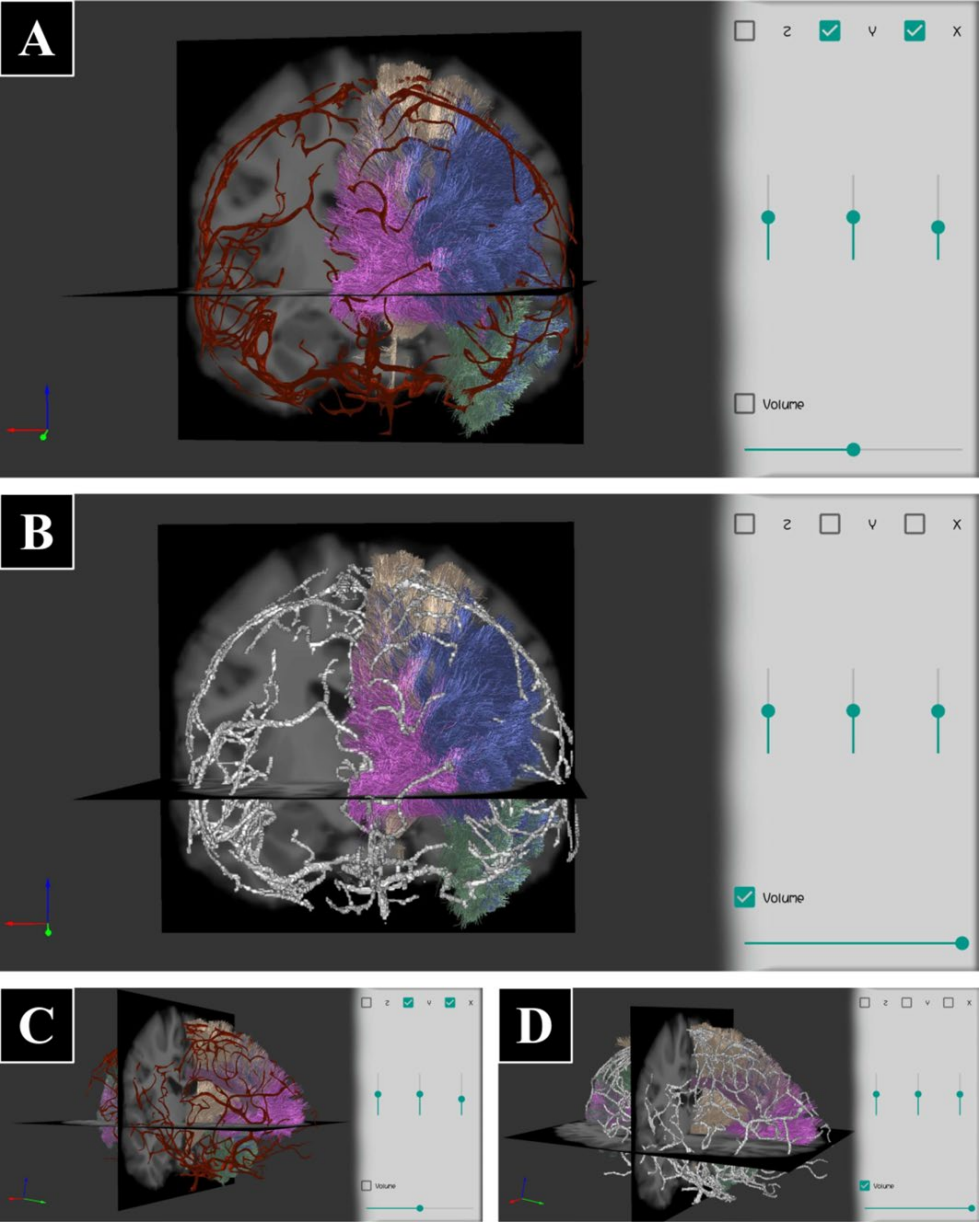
Example of brain data, including a brain MRI (skull stripped), a tractography dataset with labeled bundles, a mask of segmented arteries from MRI angiography and an arteries mesh (Dataset IV). A Coronal and axial slices of the brain image, the tractography displayed with random colors, and the arteries mesh in red. B The same slice and tractography display as in A, but with a volume rendering of the arteries mask, instead of the mesh (with maximum alpha). C, D The same as A and B, respectively, but from a sagittal oblique view
